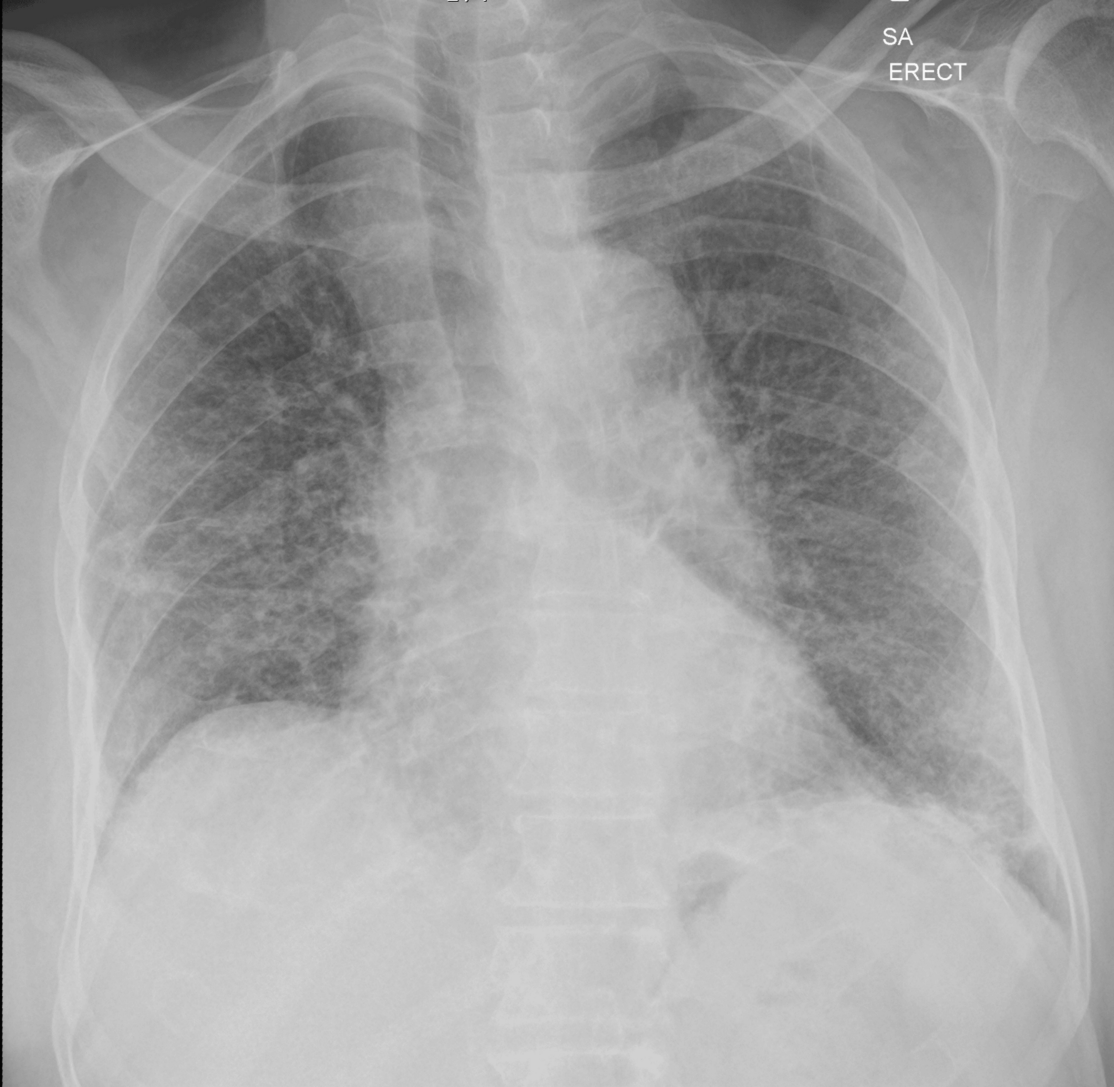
Chest X-ray showing diffuse bilateral fibrotic changes in the lungs
